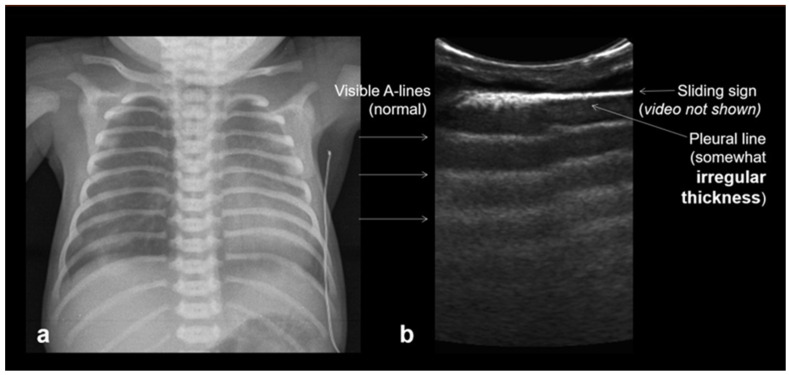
Normal to mild RDS: by chest X-ray (a); by LUS (b).
